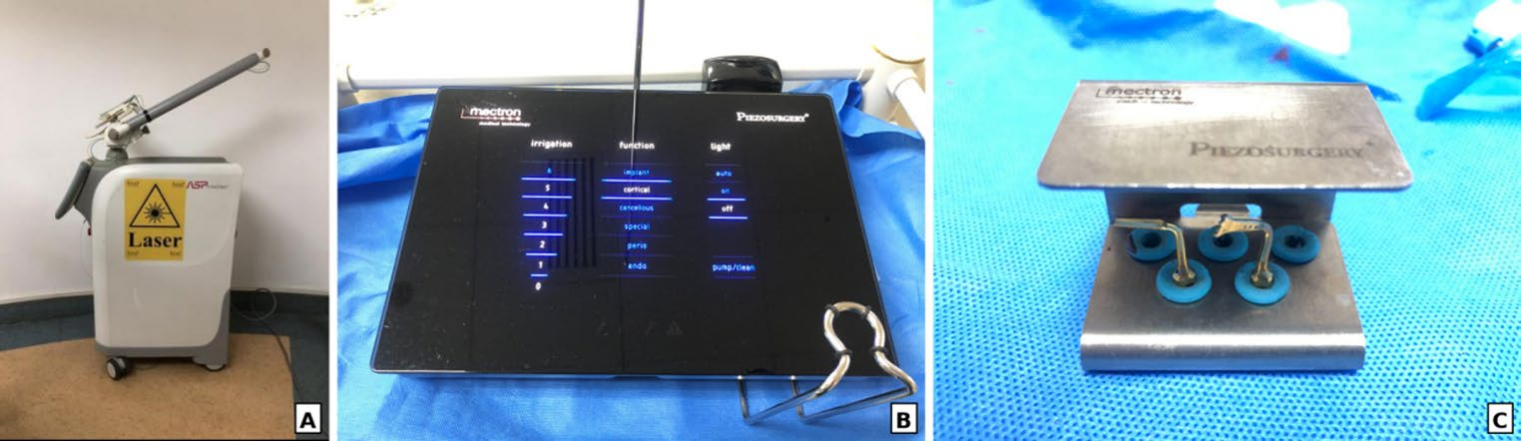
Dual-wavelength laser and ultrasonic osteotomy systems. A, Er: YAG & Nd: YAG laser unit (Fotona Light Walker). B, Piezoelectric bone surgery console (Mectron Piezosurgery). C, Piezoelectric bone surgery handpieces and tips set
